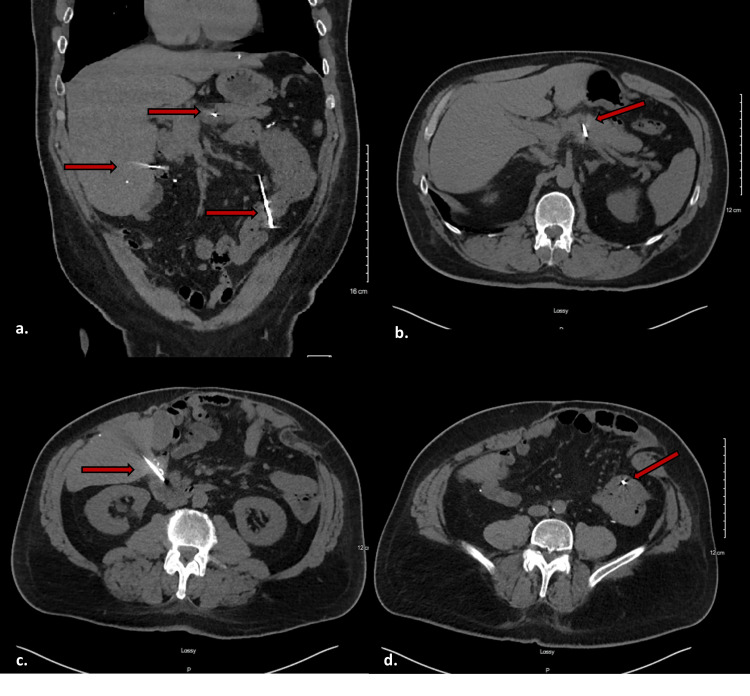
CT scan of the abdomen and pelvis showing nail locations a) Three nails seen within the abdominal cavity (red arrows); b) Nail traversing the left lobe of the liver and the middle of pancreas (red arrow). c) Nail traversing the right lobe of the liver and abutting the duodenum (red arrow). d) Nail either within or between the small bowel and mesentery (red arrow).
